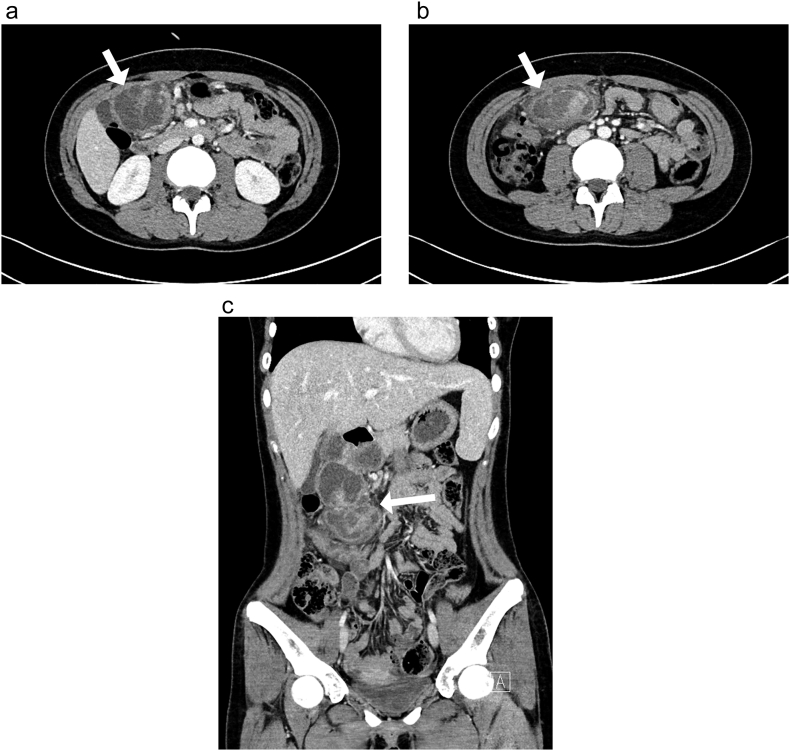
Contrast-enhanced abdomen-pelvis computed tomography. (A), (B) Axial view. (C) Coronal view. The mass on the right side of the greater omentum, abutting the left liver, gallbladder, and duodenal bulb (arrow).
